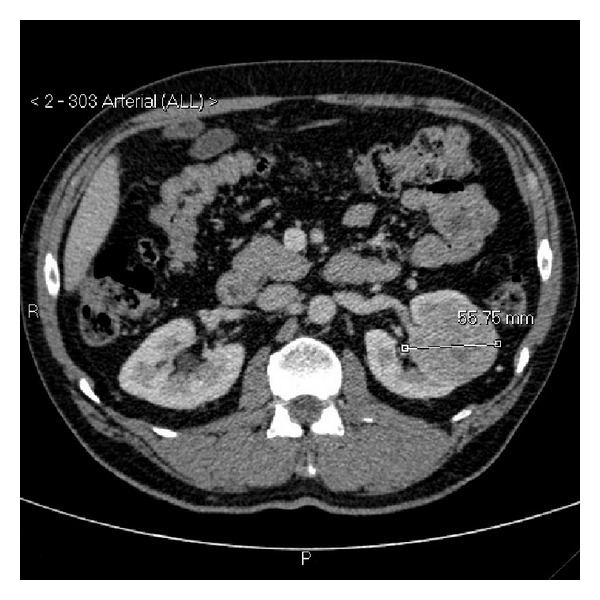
Axial portal venous phase CT scan at initial presentation demonstrating a 56 mm mass of the interpolar left kidney. Normal left renal vein.
